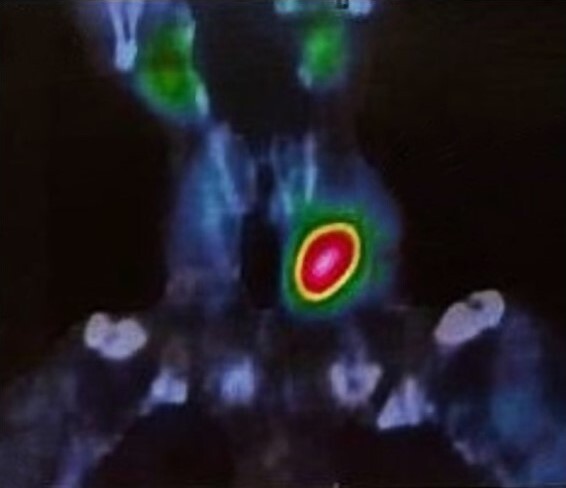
T-99m SPECT-CT shows increased tracer uptake in left lower lobe of thyroid.
